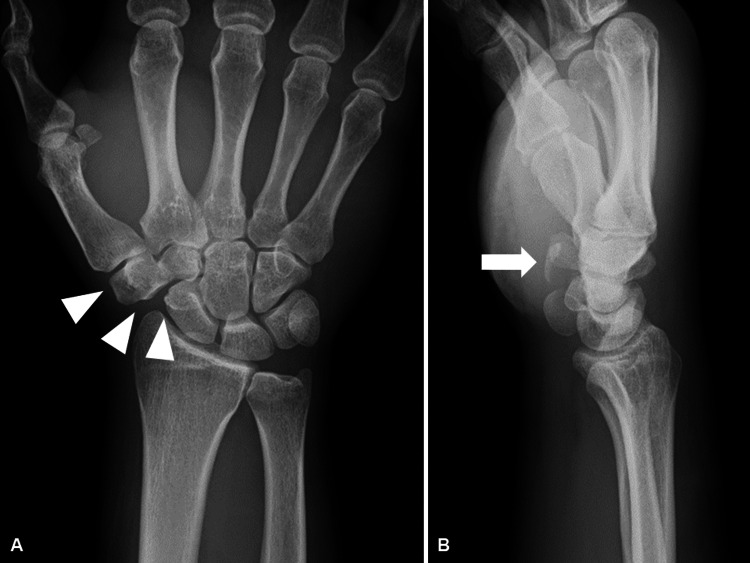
Preoperative plain radiography. (A) Anteroposterior and (B) lateral views of the right wrist (arrowheads show dislocation of the trapezium, and arrow shows volar fragment of the trapezium).
